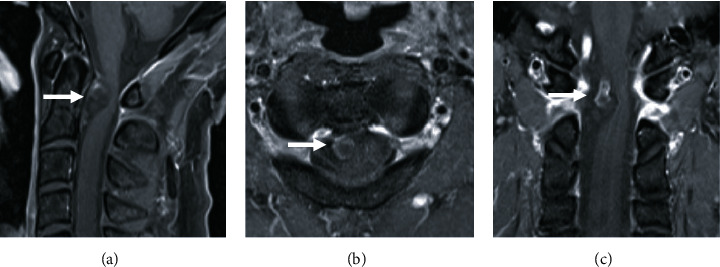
Contrast-enhanced magnetic resonance imaging of the cervical spine. Because a cyst was partially enhancing, a tumor lesion was not identifiable ((a) sagittal, (b) horizontal, (c) coronal, arrow).
